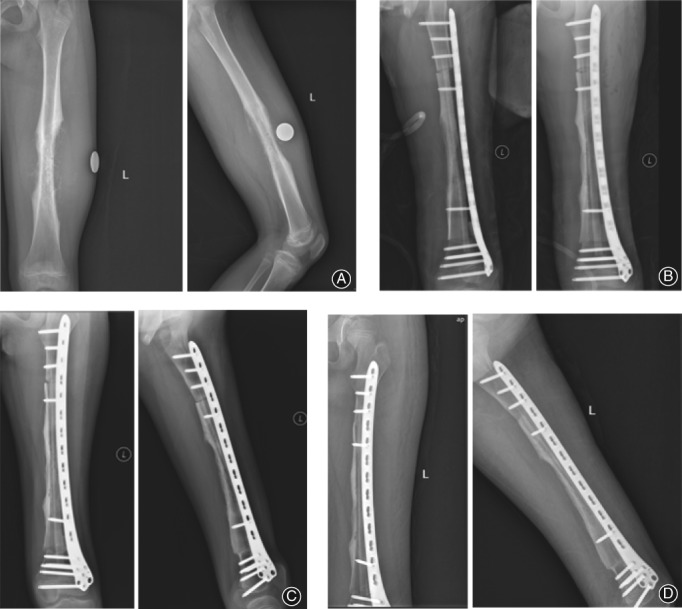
(A) The radiographs of a 13‐year‐old boy (case 7) with osteosarcoma of the left femur are shown. (B) Radiographs taken 1 day postoperatively are shown. (C) Radiographs taken 12 months postoperatively are shown. (D) Radiographs taken 27 months postoperatively are shown. Bone union and incorporation were achieved.
